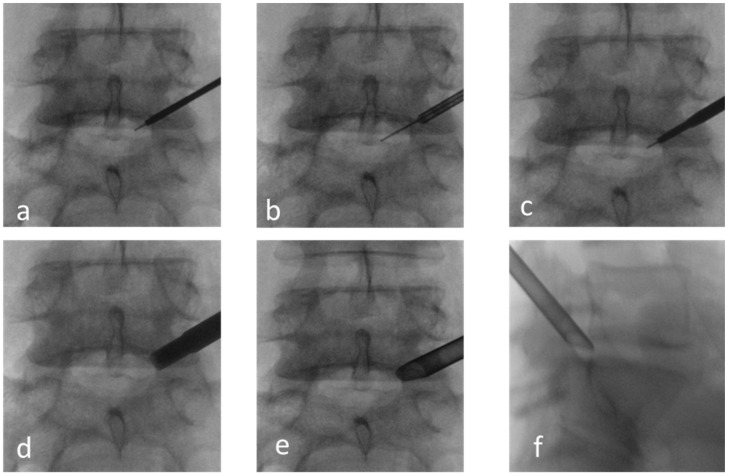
Approach to the spinal canal through foramina. (a–d) Insertion of a guide wire, dilators, and reamers into the foramina. (e,f) X-ray checking of working channel position.
